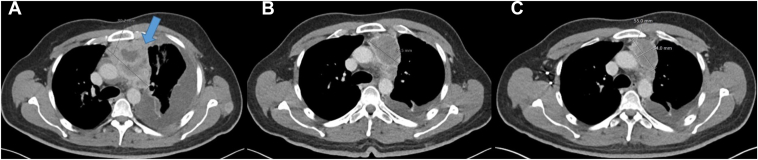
Radiologic results before and after initiation of Lenvatinib. (*A*) Contrast-enhanced CT scan of the thorax revealing a large, heterogeneous anterior mediastinal mass, denoted by arrow, before initiation of lenvatinib (baseline CT May 2023). (*B*) Contrast enhanced CT scan revealing PR 2 months post initiation of lenvatinib (July 2023). (*C*) Contrast enhanced CT scan revealing ongoing response to lenvatinib (September 2023). CT, computed tomography, PR, partial response.
